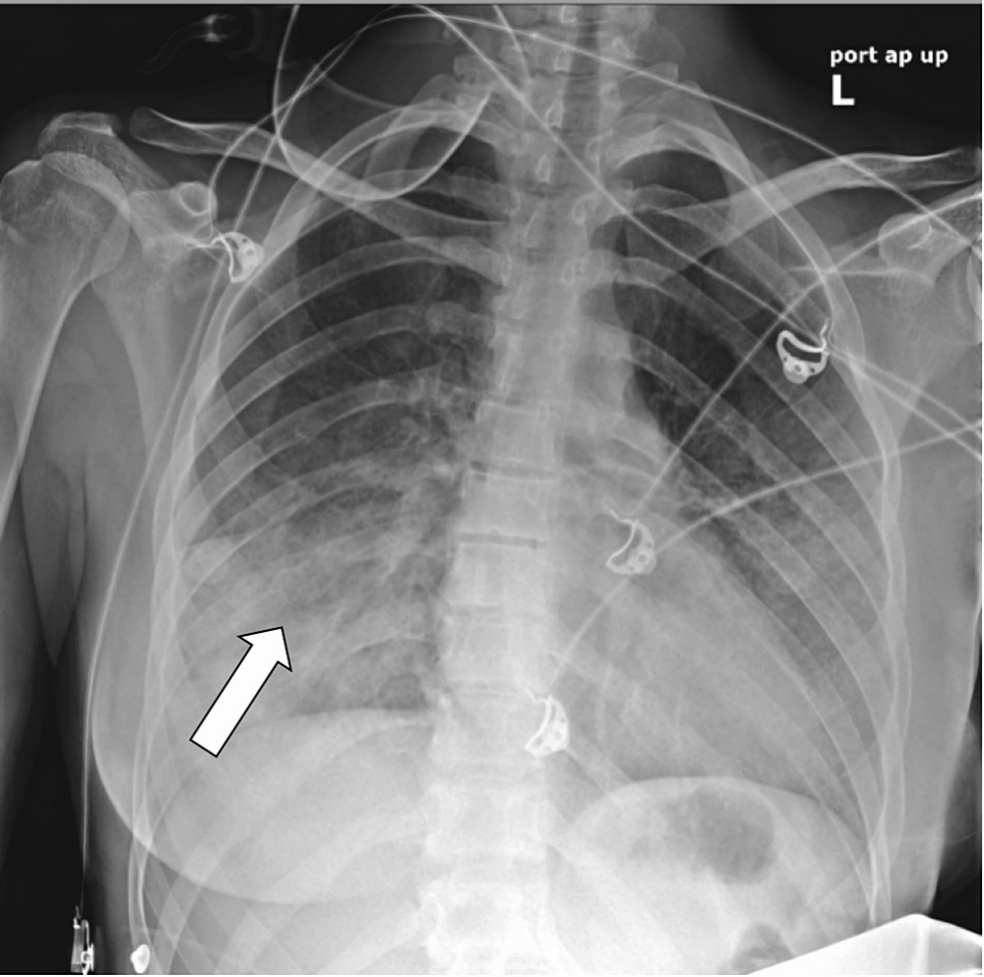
Chest X-ray showing confluent airspace disease, most prominent at the right lower lobe (arrow)
